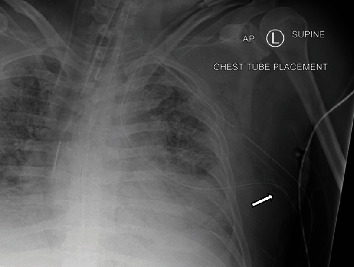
Postintervention chest X-ray showing improved kinking in chest tube #1 and fully expended lung in a patient with COVID-19 respiratory failure on mechanical ventilation.
